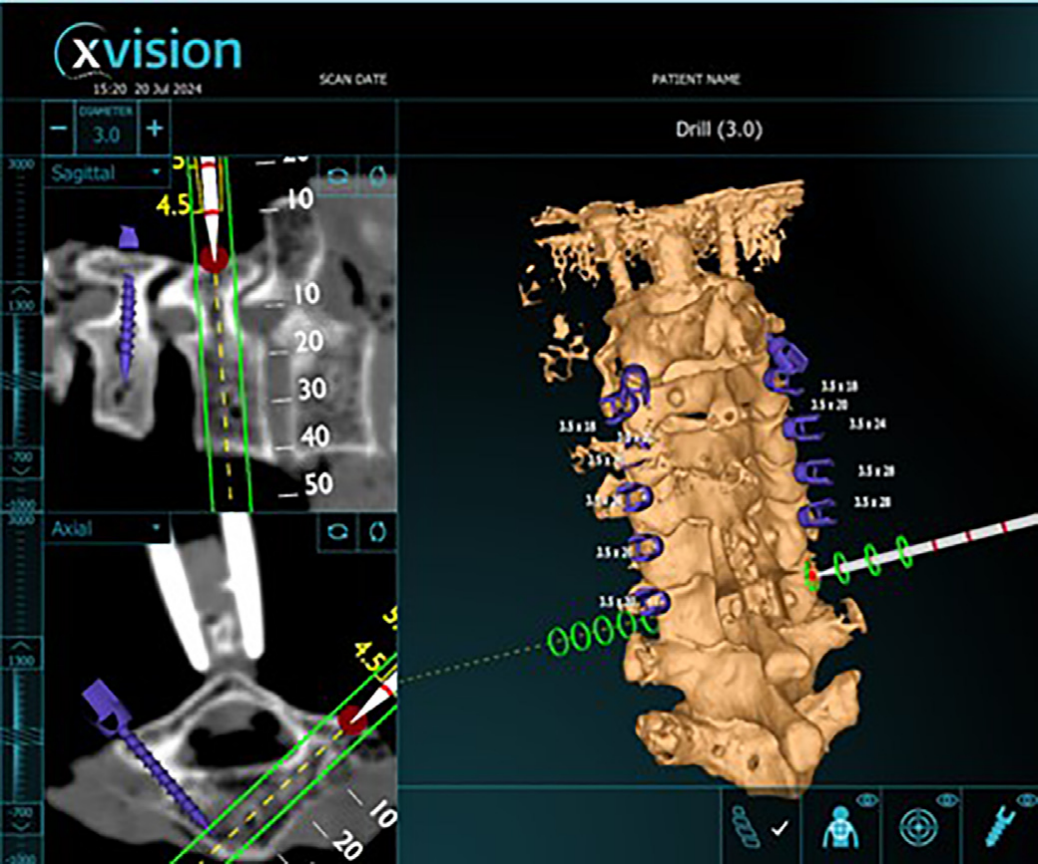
Screen shot showing initial trajectory on axial CT scan, sagittal CT scan and 3D model
